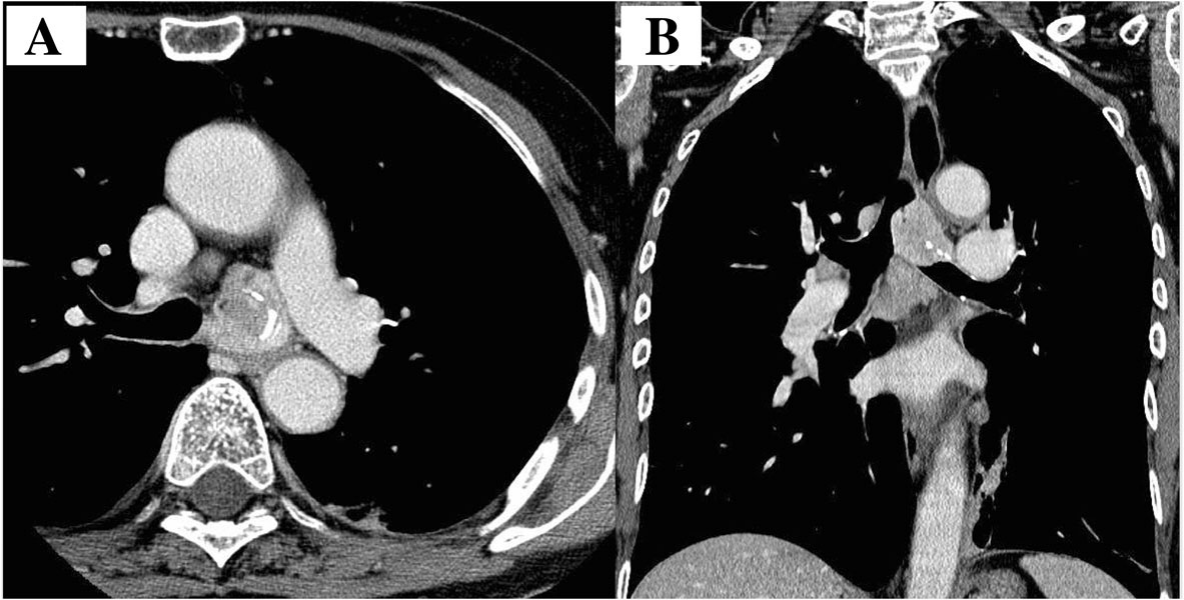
Computed tomography findings. a Coronal and b Sagittal view. A solid left main bronchial tumor with carinal involvement is observed
